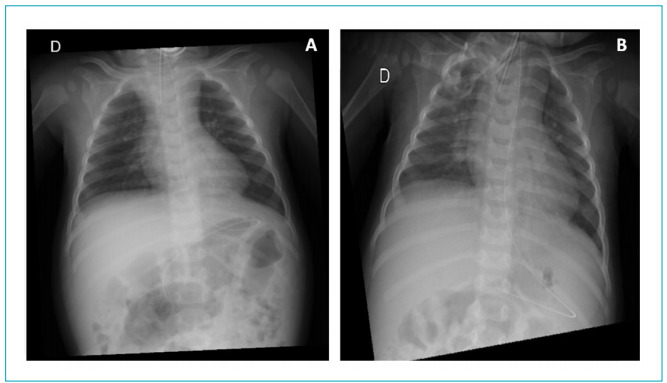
Chest radiography: (A) without changes; (B) perihilar infiltrate and apparent middle lobe consolidation.
